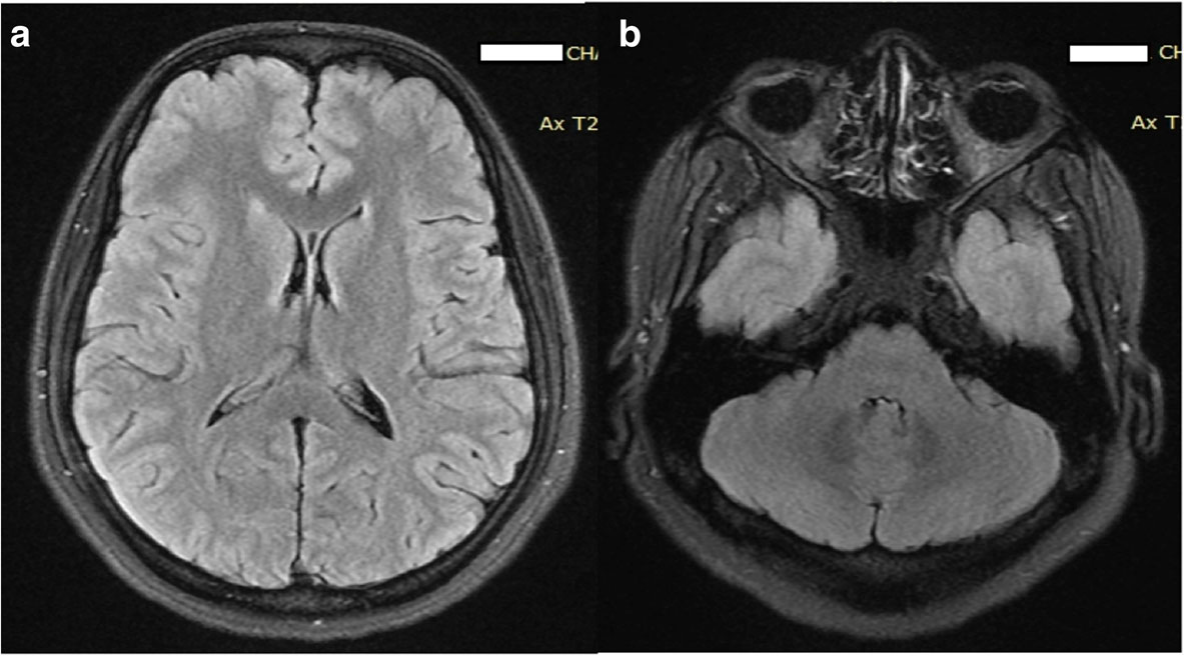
MRI images with FLAIR sequence showing normal cerebellum
